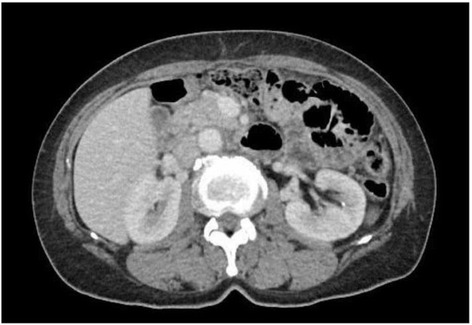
Follow-up CT in 18 months later showed marked regression of pancreatic desmoid tumor under celecoxib treatment
